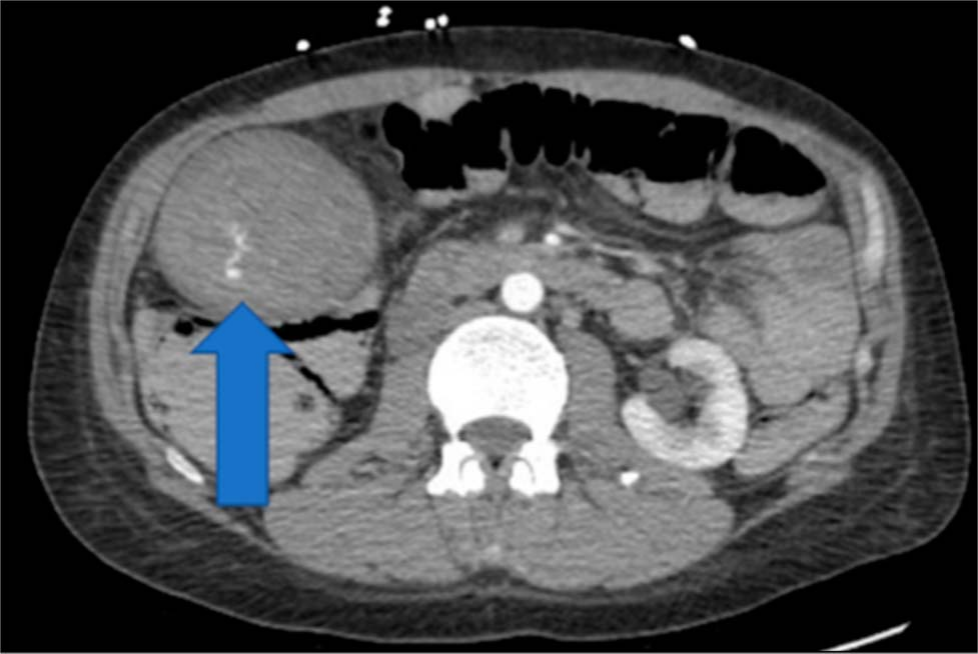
CT angiography (CTA) of the abdomen in axial view demonstrated a markedly distended gallbladder filled with a hematoma and small foci of arterial hemorrhages at the blue arrow.
